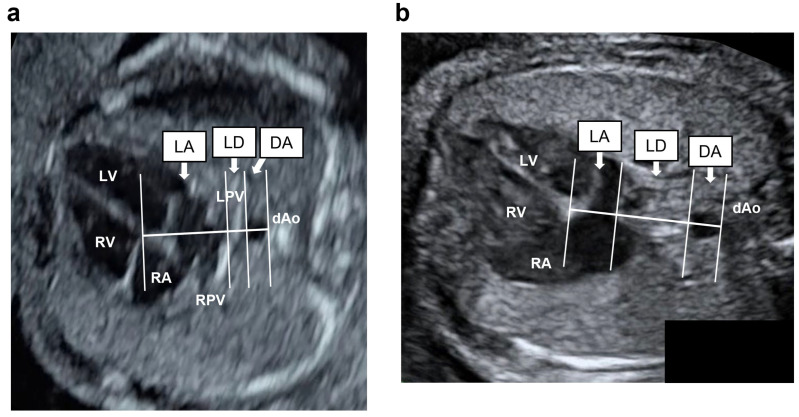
Assessment of the PLAS index and LAPSD ratio on the four-chamber view in a normal case (a) and a case of TAPVC (b). Both biometric parameters are based on the characteristic ultrasound finding that LD is wider in TAPVC (b) than in the normal case (a). PLAS index, post-left atrium space index; LAPSD ratio, left-atrial posterior-space-to-diagonal ratio; LA, crux–posterior left atrial wall distance; LD, posterior left atrial wall–descending aorta distance; DA, diameter of the descending aorta; LV, left ventricle; RV, right ventricle; RA, right atrium; LPV, left pulmonary vein; RPV, right pulmonary vein; dAo, descending aorta.
